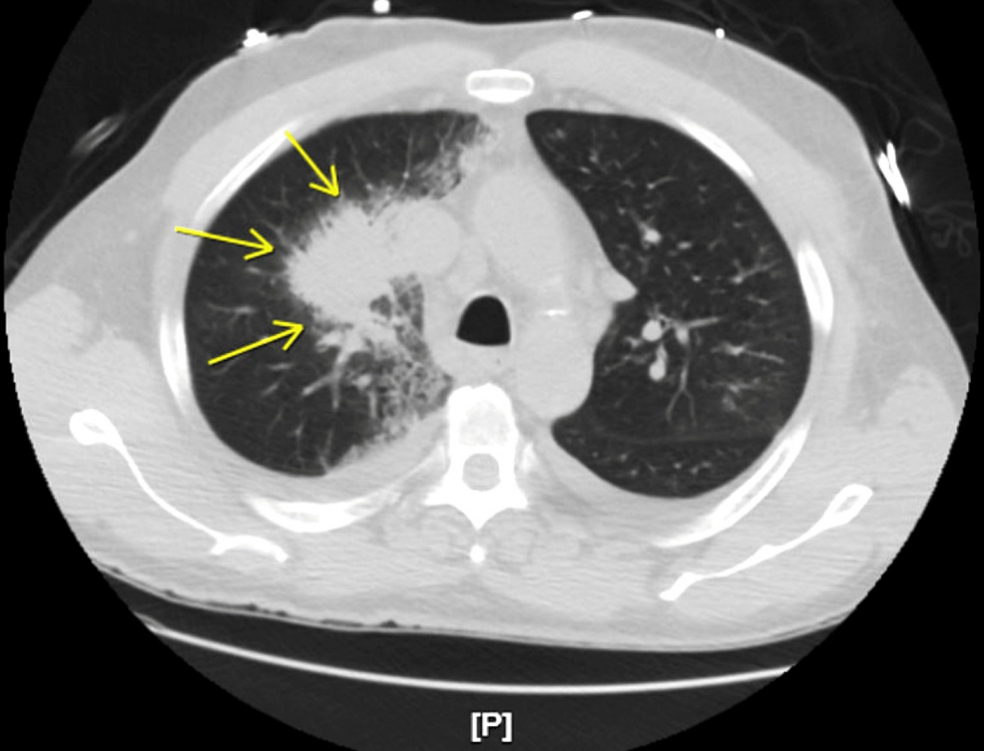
CT scan showing a lung mass sized 6.3×4.7×3.2 cm and bilateral small pleural effusion.
